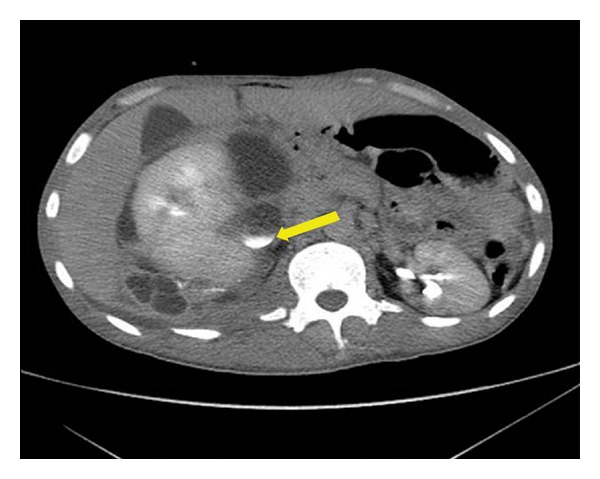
CT scan showing urinoma, contrast extravasation (arrow) with a right pelvicaliceal dilatation.
